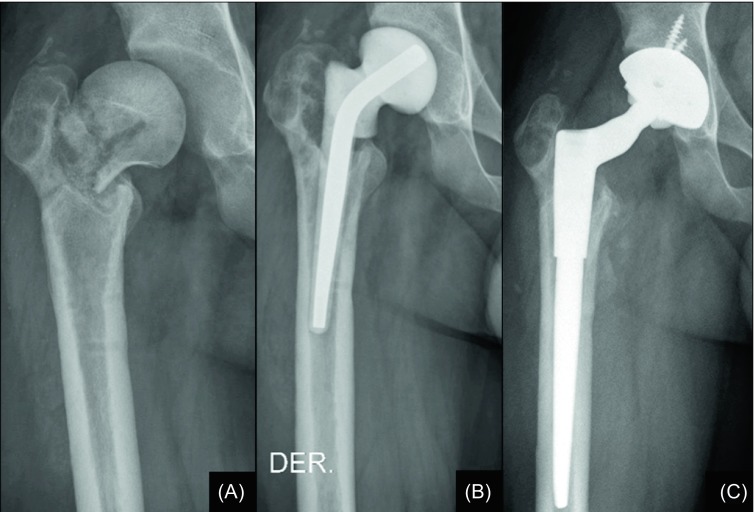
(A) Anteroposterior right hip radiograph of a 25-year old male with sequelae of a traumatic basicervical right hip fracture treated at another institution one year earlier, evidencing signs of non-union. (B) Anteroposterior X-ray view of the same patient after treatment with an antibiotic-impregnated cement spacer, as part of a two-stage protocol revision surgery. (C) Radiographic image of the same hip eight weeks later, following reimplantation with a distally fixed uncemented stem and a cementless acetabular component.
